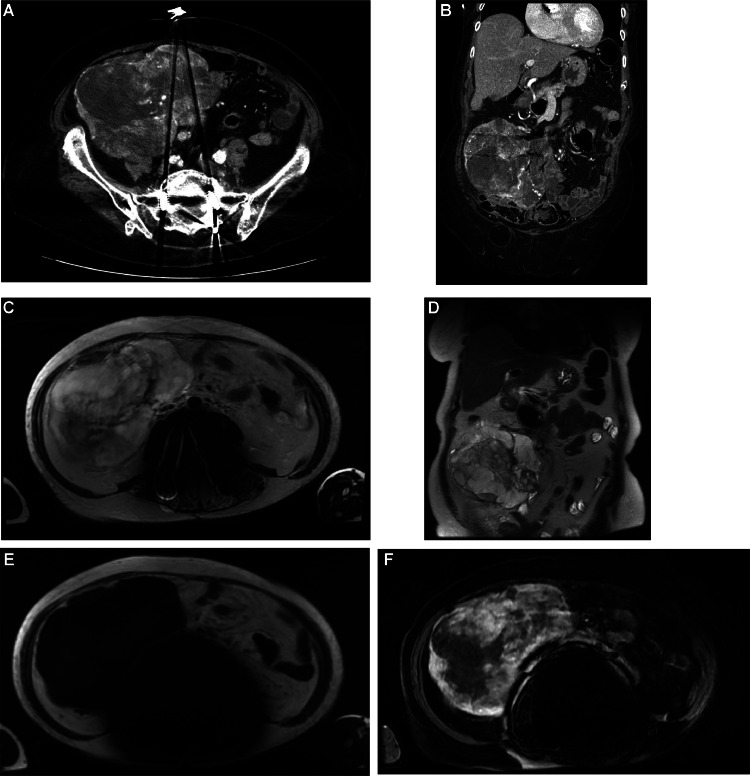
(A and B) Axial & coronal CT abdomen/pelvis demonstrating a large heterogeneous partially vascular and necrotic mass measuring 11.0 × 15.7 × 15.5 cm within the right lower quadrant of the abdomen/mesentery. The mass extends from the anterior pararenal space and compresses the ascending colon anteriorly. (C and D) Axial & coronal T2 weighted MRI abdomen showing diffuse heterogeneous hyperintense signal. (E and F) Axial T1 & T1 weighted fat saturated postcontrast MR images demonstrating prominent peripheral enhancement of the mass.
